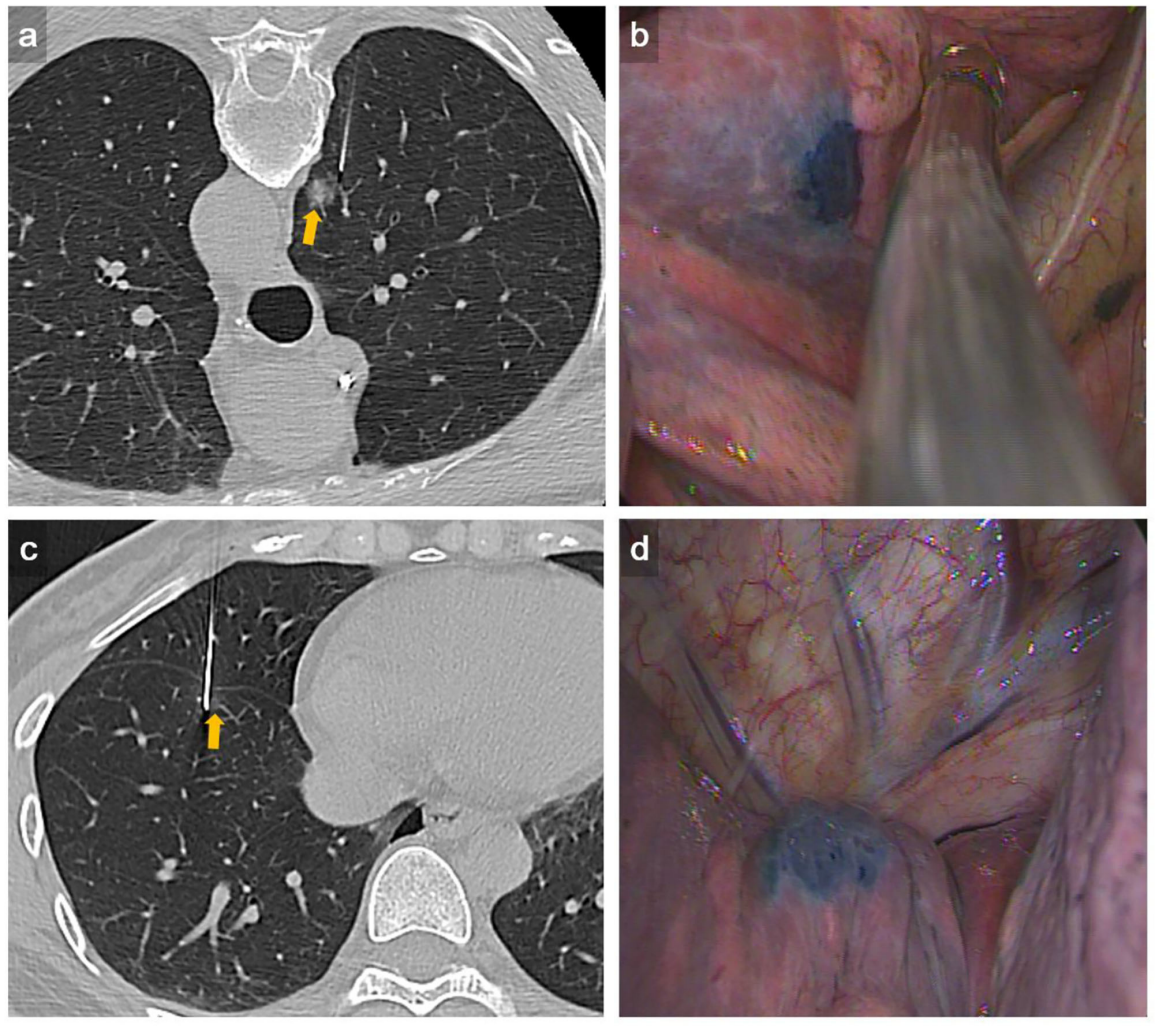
Representative example of complex dye localization. (a) An axial CT imaging with lung window during localization showed a 22-gauge Chiba needle introduced tangentially to the RUL part-solid lung nodule (arrow). (b) VATS imaging showed deposition of methylene blue on the RUL lung surface seen on the visceral pleura. The pathology result revealed adenocarcinoma. (c) An axial CT imaging with lung window during localization showed a 22-gauge Chiba needle introduced to the RLL GGN using trasfissural approach (arrow). Using this method, a total of three times of pleural puncture was done. (d) VATS imaging showed deposition of methylene blue on the RLL lung surface seen on the visceral pleura adjacent to right major fissure. The pathology result revealed AAH.
